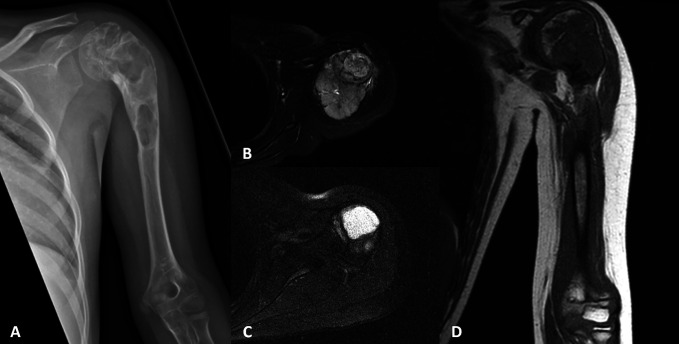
Preoperative radiographs (A) showing a proximal humeral Ewing sarcoma. Before the induction of chemotherapy, the patient had a large soft-tissue mass (B) on T2-weighted MRI, which resolved before surgery (C). After chemotherapy on coronal T1-weighted MRI (D), there is marrow replacement in the physis after chemotherapy.
